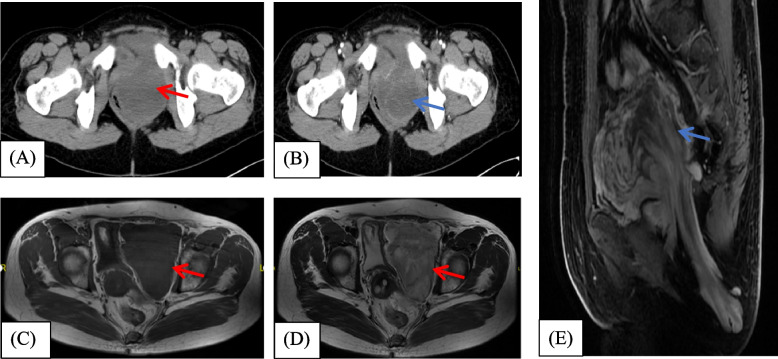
CT images of AAM (case11) The lesion was located in the left para-rectal space (Red arrow). A Axial plain CT scan showed lower density of the lesion compared with density of the muscle. B Axial enhancement CT scans showed the lesions are mildly enhanced and appear ‘layered’ (Blue arrow). The mass was clearly bounded, and the rectum and uterus were displaced to the right. MRI images of AAM (case7) A soft tissue mass was seen in the left pelvis with the lesion extending through the pelvic floor to the subcutaneous fat of the left buttock. The lesion was well defined and the surrounding structures were displaced (Red arrow). C Axial unenhanced T1-weighted MRI shows a mass with isosignal relative to the muscle. D Axial T2-weighted MRI showed the mass as a mixed signal with predominantly high signal. E Sagittal enhanced T1-weighted MRI showed the swirling and layering pattern of marked enhancement within the mass (Blue arrow)
